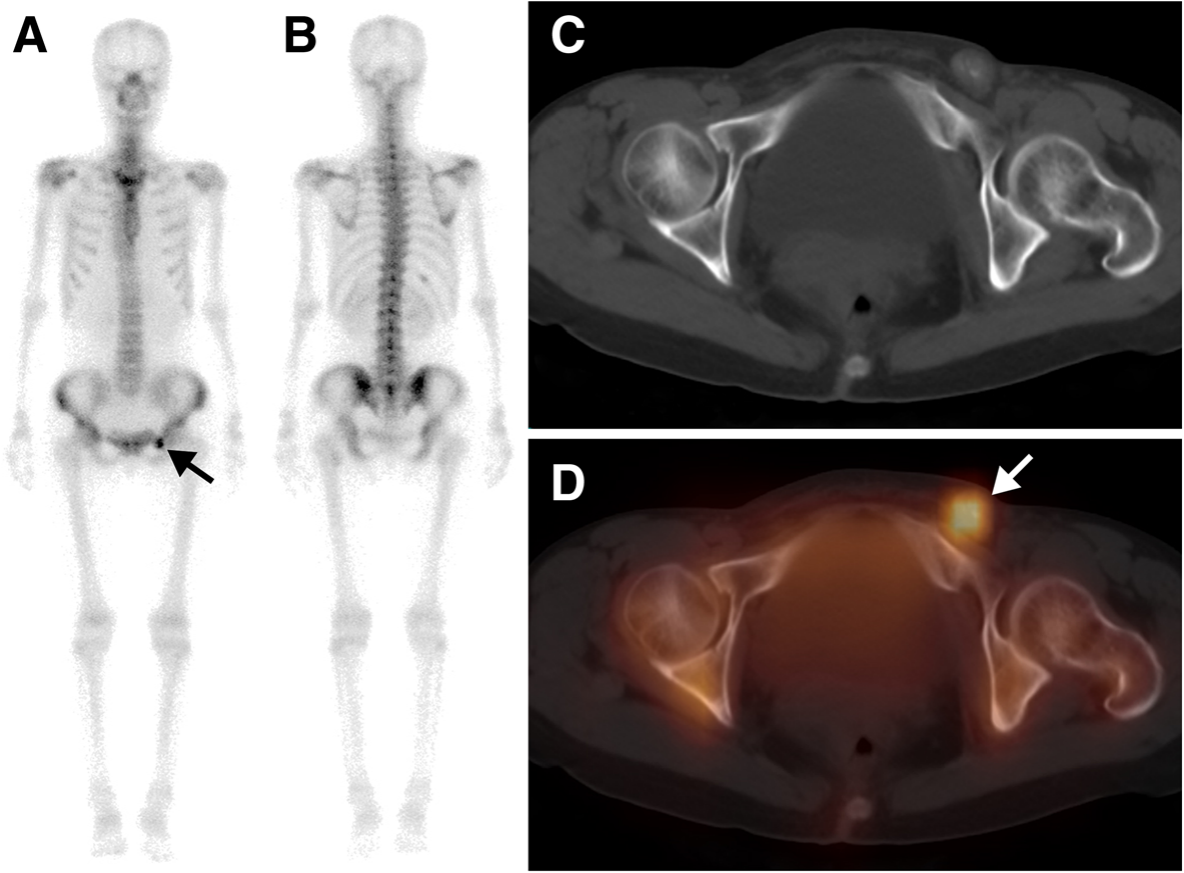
A 40-year-old woman with ovarian cancer (papillary adenocarcinoma) after surgery and chemotherapy for 2 years, who was referred for routine follow-up. The whole-body scan (a, anterior; b, posterior) revealed a single focus of high ^99m^Tc-MDP activity in the left pubic bone region (black arrow). Axial CT (c), and SPECT/ CT images (d) showed that the elevated ^99m^Tc-MDP activity was located in a left inguinal lymph node with calcification (white arrow). Resection of the lymph node was performed and pathologic analysis confirmed the diagnosis of lymph node metastases
